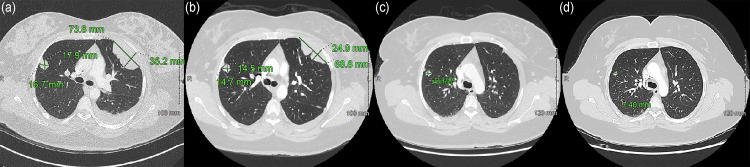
Chest CT demonstrating (a) two initial pulmonary masses, (b) post-cycle 3, (c) post-cycle 6, and (d) post-cycle 12 changes to pulmonary masses.
